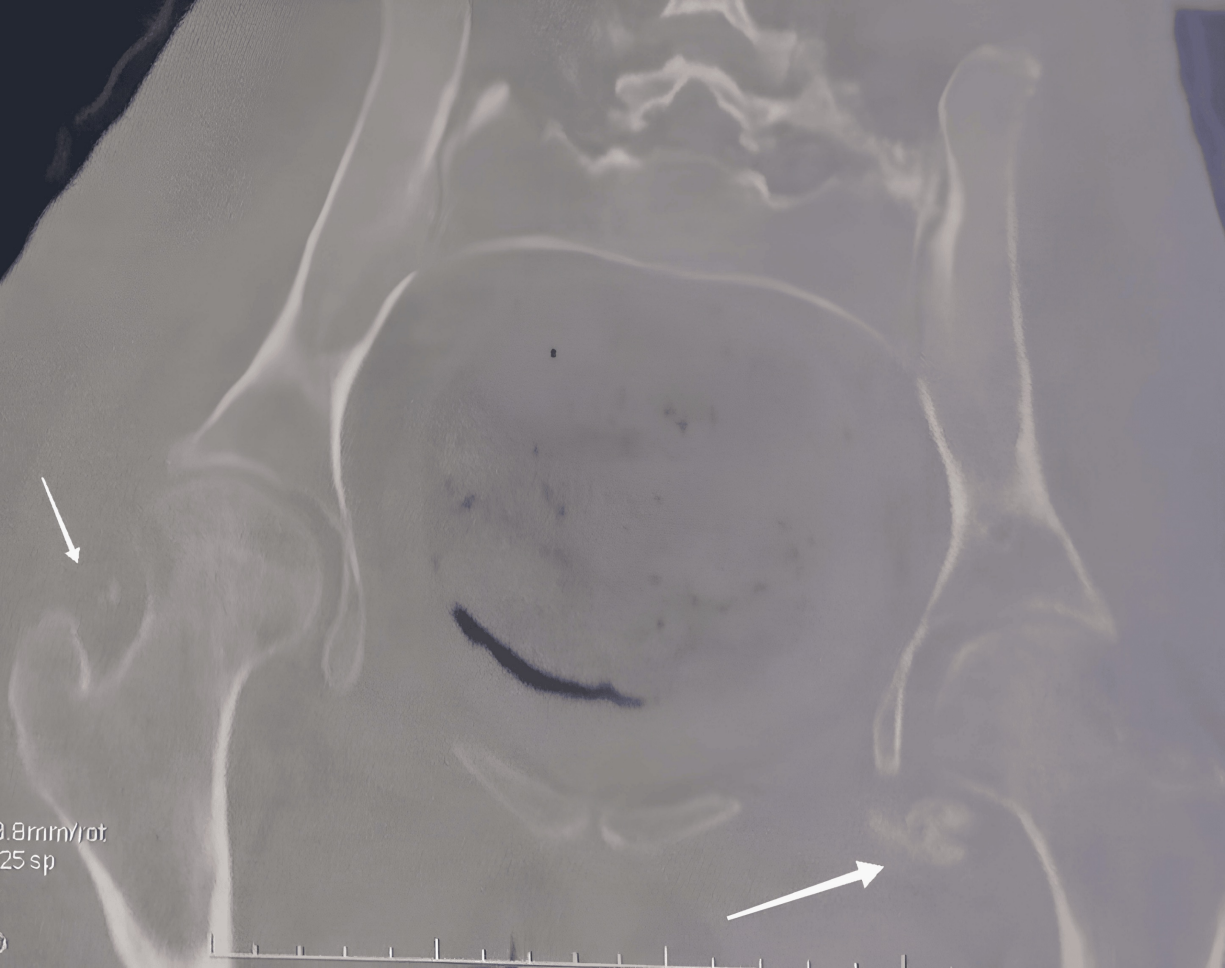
Coronal CT scan of the pelvis This image confirms the presence of bilateral HOs (indicated by white arrows) in the soft tissues around the hip joints, particularly visible anteriorly. HOs: Heterotopic ossifications
